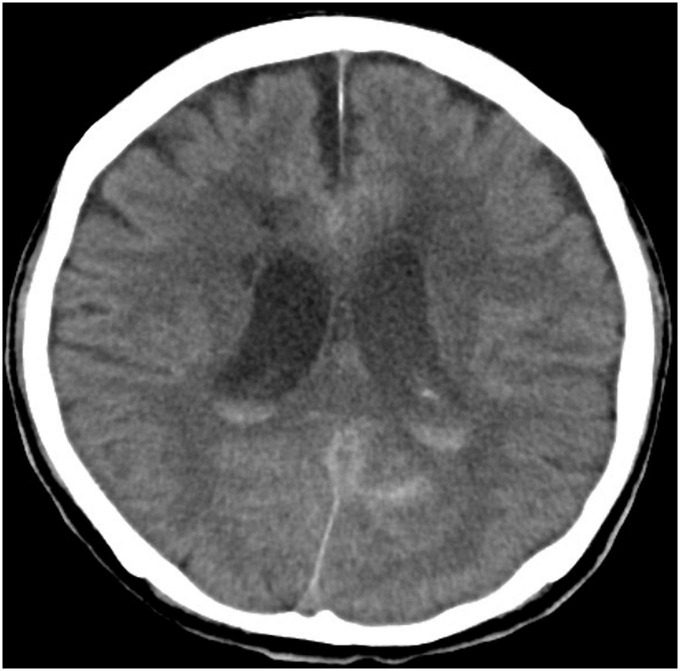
Computed tomography brain showing intraventricular extension leading to hydrocephalus.
